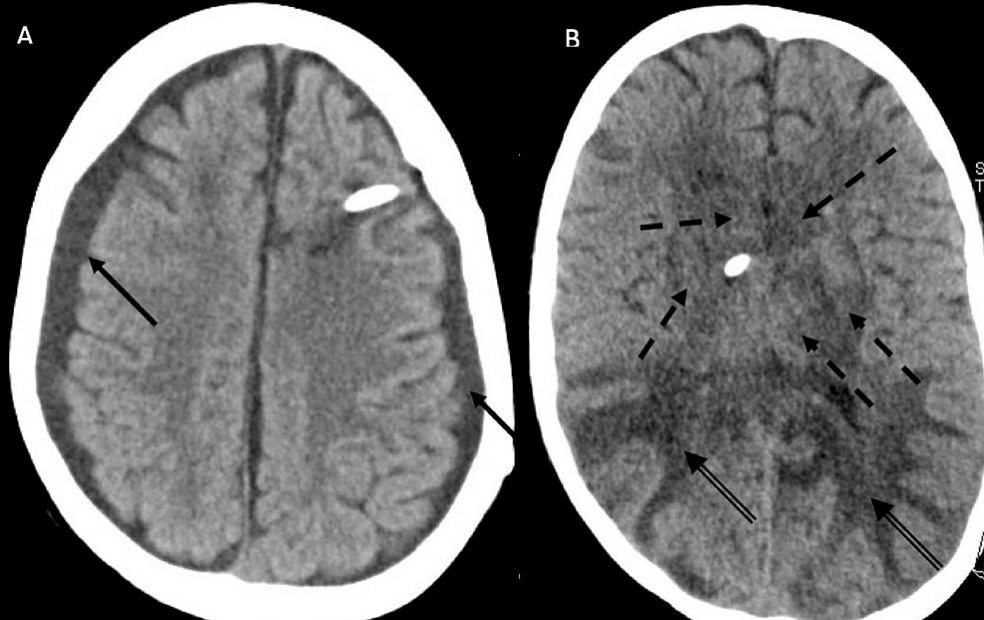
Non-enhanced CT scan of the brain. (a) Bilateral subdural effusion (arrows) secondary to over shunting. (b) Follow-up images revealed interval development of bilateral predominately parieto-occipital white matter hypodensities (double arrows) suggestive of PRES, poor differentiation of the basal ganglia and thalami with discrete hypodensities (dashed arrows), concerning hypoxic-ischemic encephalopathy, collapsed ventricular system from over shunting.
